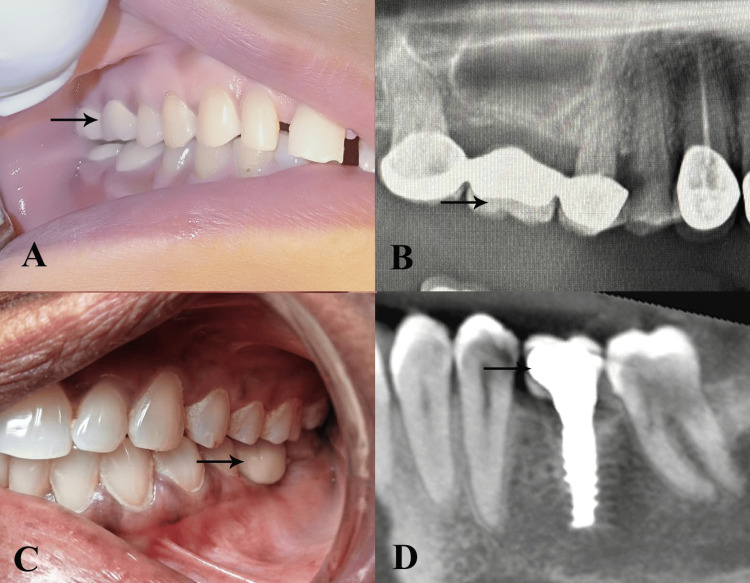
Comparison of tooth-supported three-unit fixed dental prosthesis and single-piece dental implant restoration (A) Intraoral clinical photograph showing a three-unit fixed dental prosthesis replacing a missing right maxillary first molar. The arrow indicates the pontic (false tooth) of the bridge supported by crowned abutment teeth. (B) Cone-beam computed tomography (CBCT) sagittal view of the single-piece dental implant of the mandibular left first molar. The arrow highlights the implant body surrounded by peri-implant bone, demonstrating the absence of a pontic and direct load transfer to the alveolar bone. Original intraoral images of the patients from the study, used with patients' permission.
